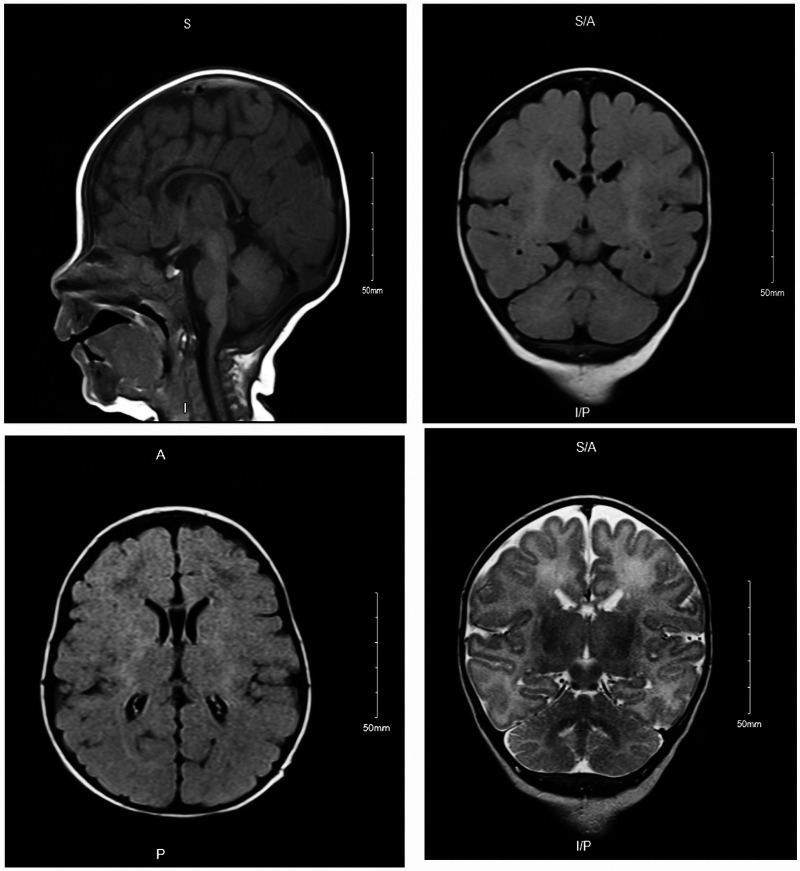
Brain MRI of affected individual (IV-2) revealed generalized volume loss manifested by a prominent ventricles and extra-axial CSF spaces. Posterior fossa structures, paranasal sinus, mastoid air cells, and visualized orbits were unremarkable.
